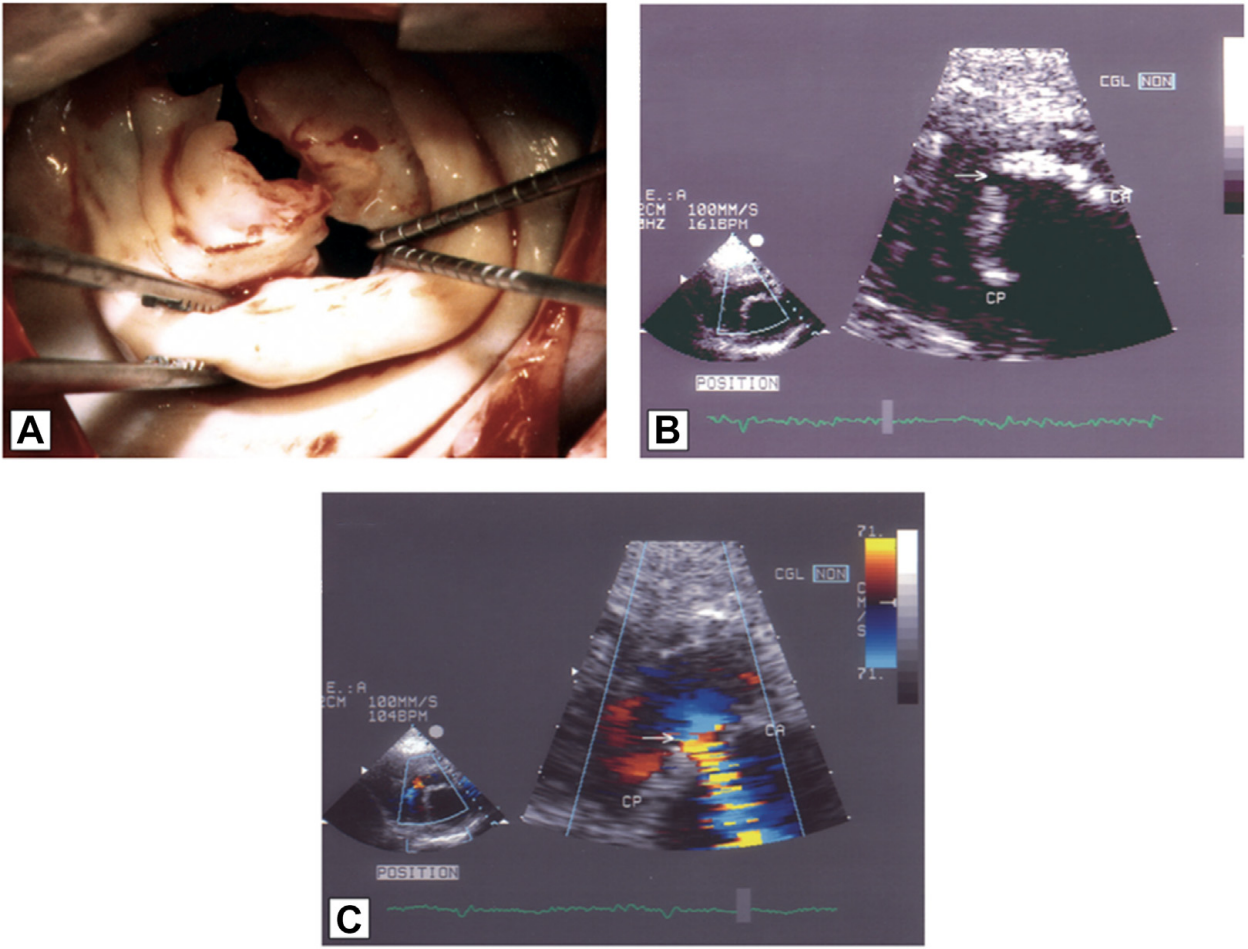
Example of Anterior Leaflet Tear Following Percutaneous Balloon Mitral Commissurotomy in a Patient With Calcified Mitral Stenosis (A) Intraoperative view showing a tear in the mid part of the anterior mitral leaflet. (B) Transthoracic echocardiography showing the tear of the anterior mitral leaflet. (C) Color Doppler confirming severe mitral regurgitation. Adapted from Vahanian A. Percutaneous balloon mitral commissurotomy. In The PCR-EAPCI Textbook, Europa Publishing.
